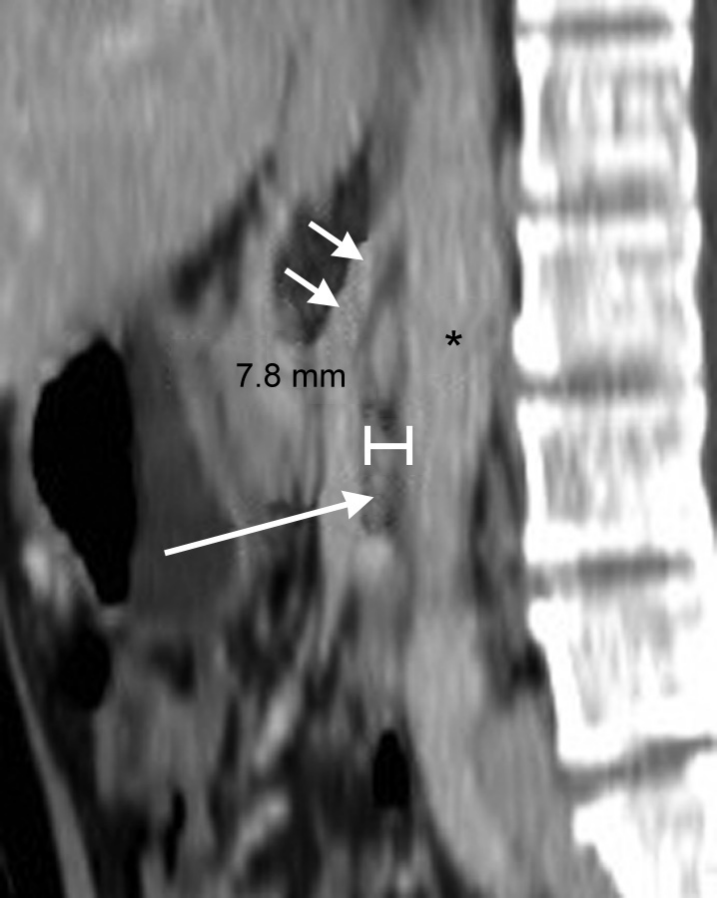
Sagittal reconstructed CT scanning of the same patient as Figure 1 shows reduced AMD of about 8 mm between SMA (double arrows) and aorta (*). Narrowed 3rd part of duodenum is seen (long arrow)
